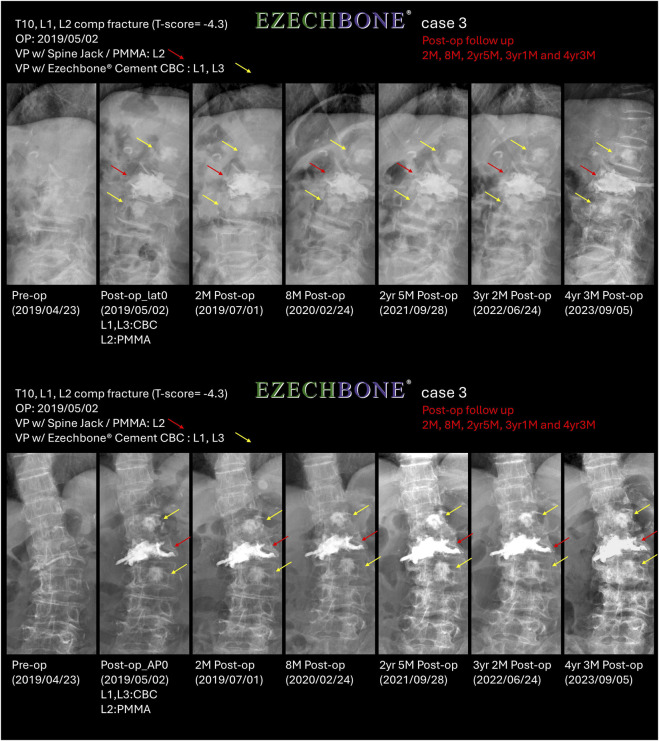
The preoperative and a series of follow-up radiographs in case 3.
